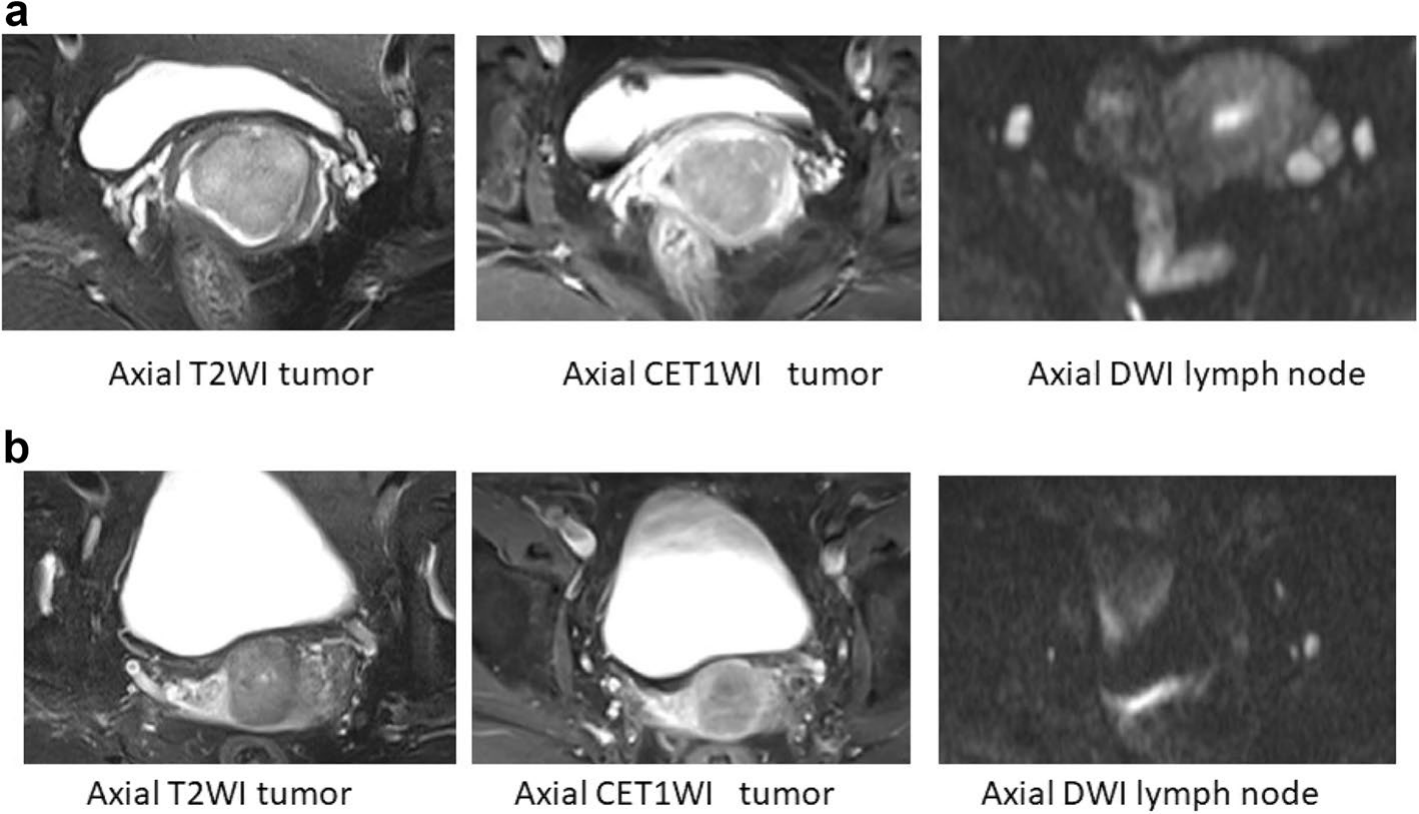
a Age, 64 years; stage, IIA2; tumour volume, 15.76 cm^3^; SCC-Ag, 2.9 ng/L; MRI-LN, 10 mm, 9 mm, true-positive; D-MIL score of T2WI tumour, 0.691, true-positive; hybrid model score, 0.778, true-positive. b Age, 53 years; stage, IIA2; tumour volume, 13.11cm^3^; SCC-Ag, 0.6 ng/L; MRI-LN, 8 mm, true- negative; D-MIL score of T2WI tumour, 0.202, true-negative; hybrid model score, 0.161, true-negative
